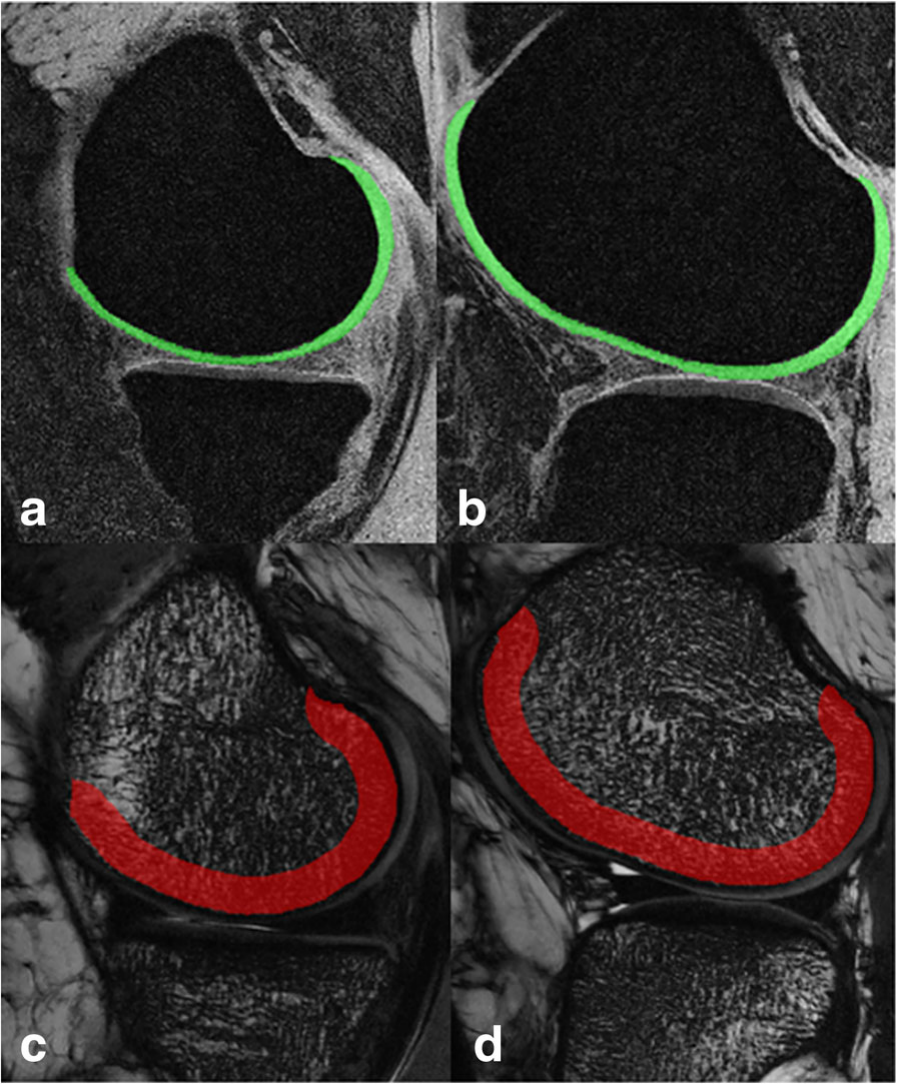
Images of the regions of interest acquired by 3T MRI. Analysis of the cartilage region in the center of the medial femoral condyle (a) and the lateral femoral condyle (b) by 3D FFE imaging and the analysis of the subchondral bone region in the center of the medial femoral condyle (c) and the lateral femoral condyle (d) by 3D BFFE imaging
